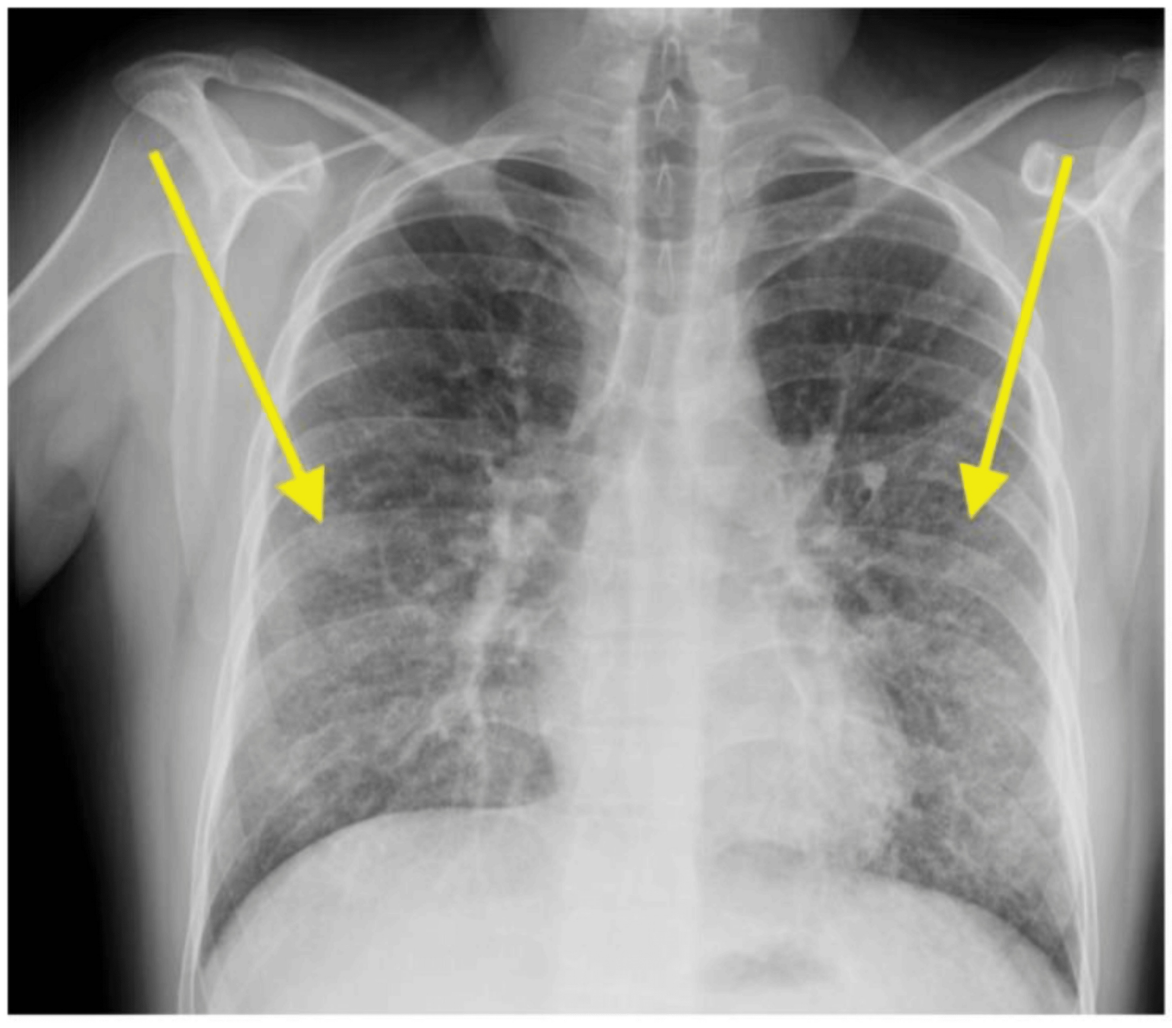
Chest radiography demonstrating diffuse bilateral mid to lower zone lung opacities (yellow arrows)
